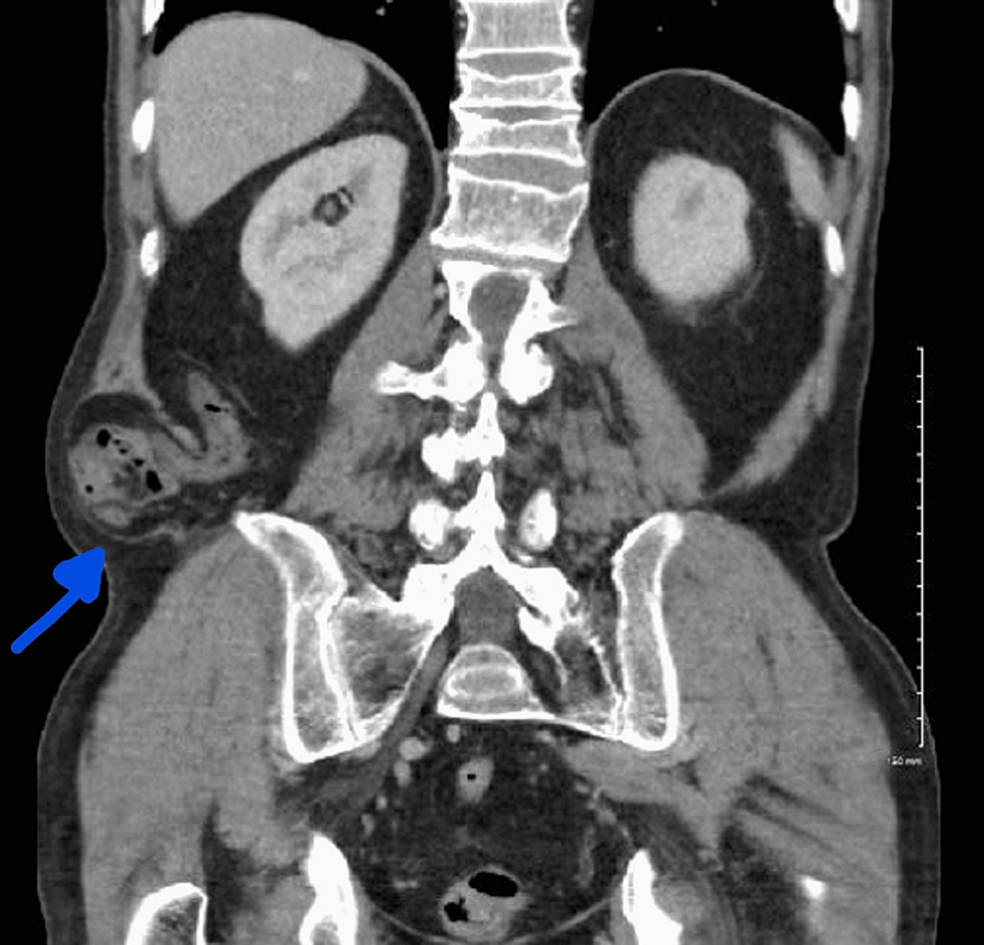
Preoperative CT of the abdomen/pelvis, coronal view, demonstrating a right lumbar defect now containing cecum (see blue arrow)
